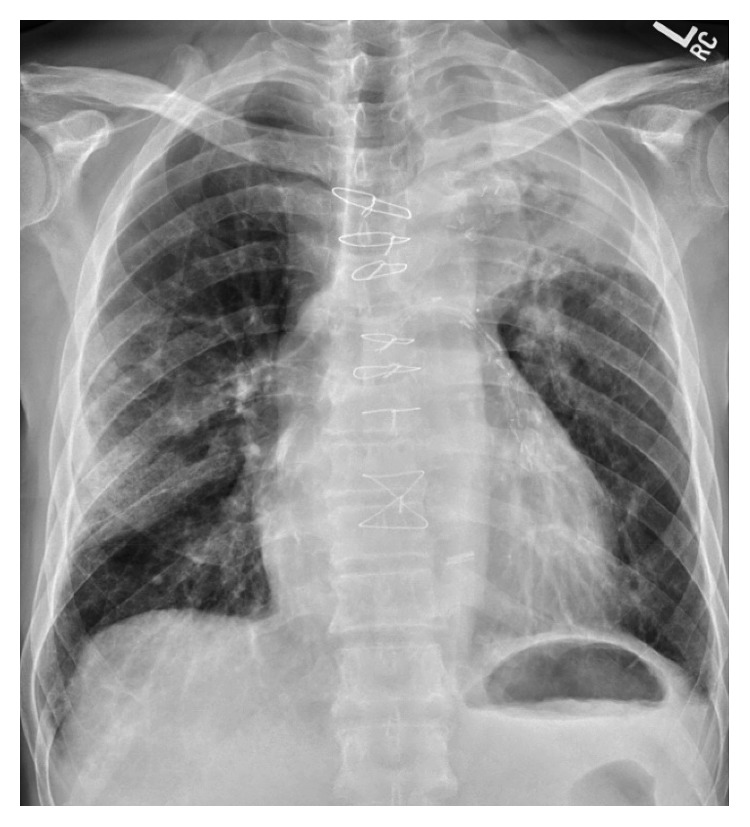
Chest radiograph on admission: multifocal pneumonia.
